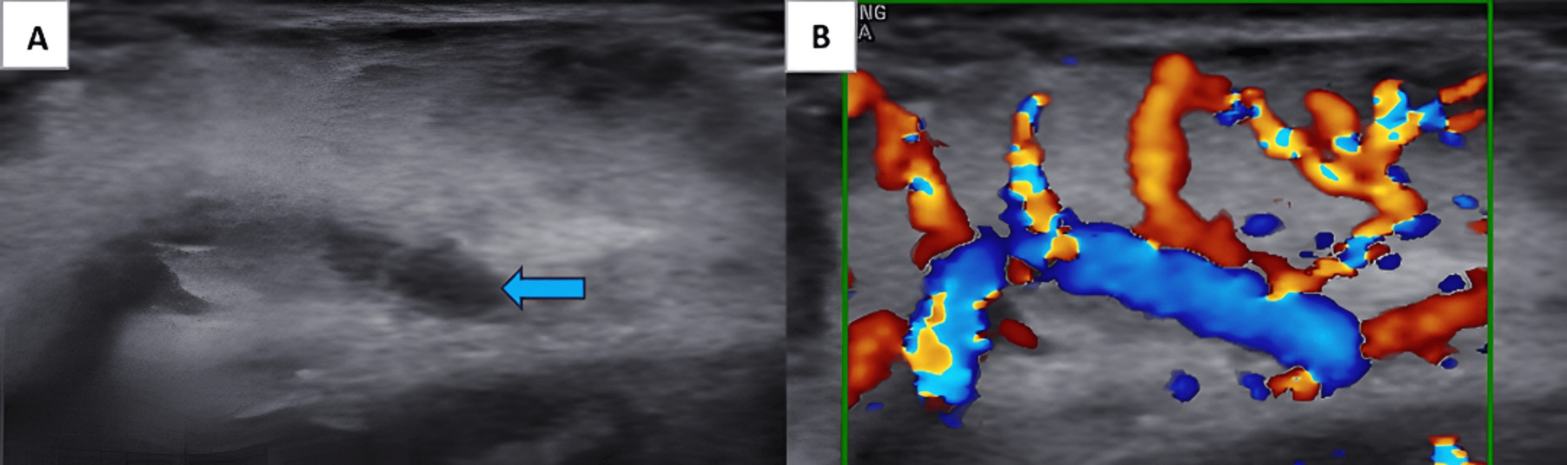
(A) B-mode USG showing predominantly hyperechoic lesion with hypoechoic tubular channels (blue arrow) within it and (B) color Doppler USG showing raised vascularity with feeding vessel noted within it USG: ultrasonography
